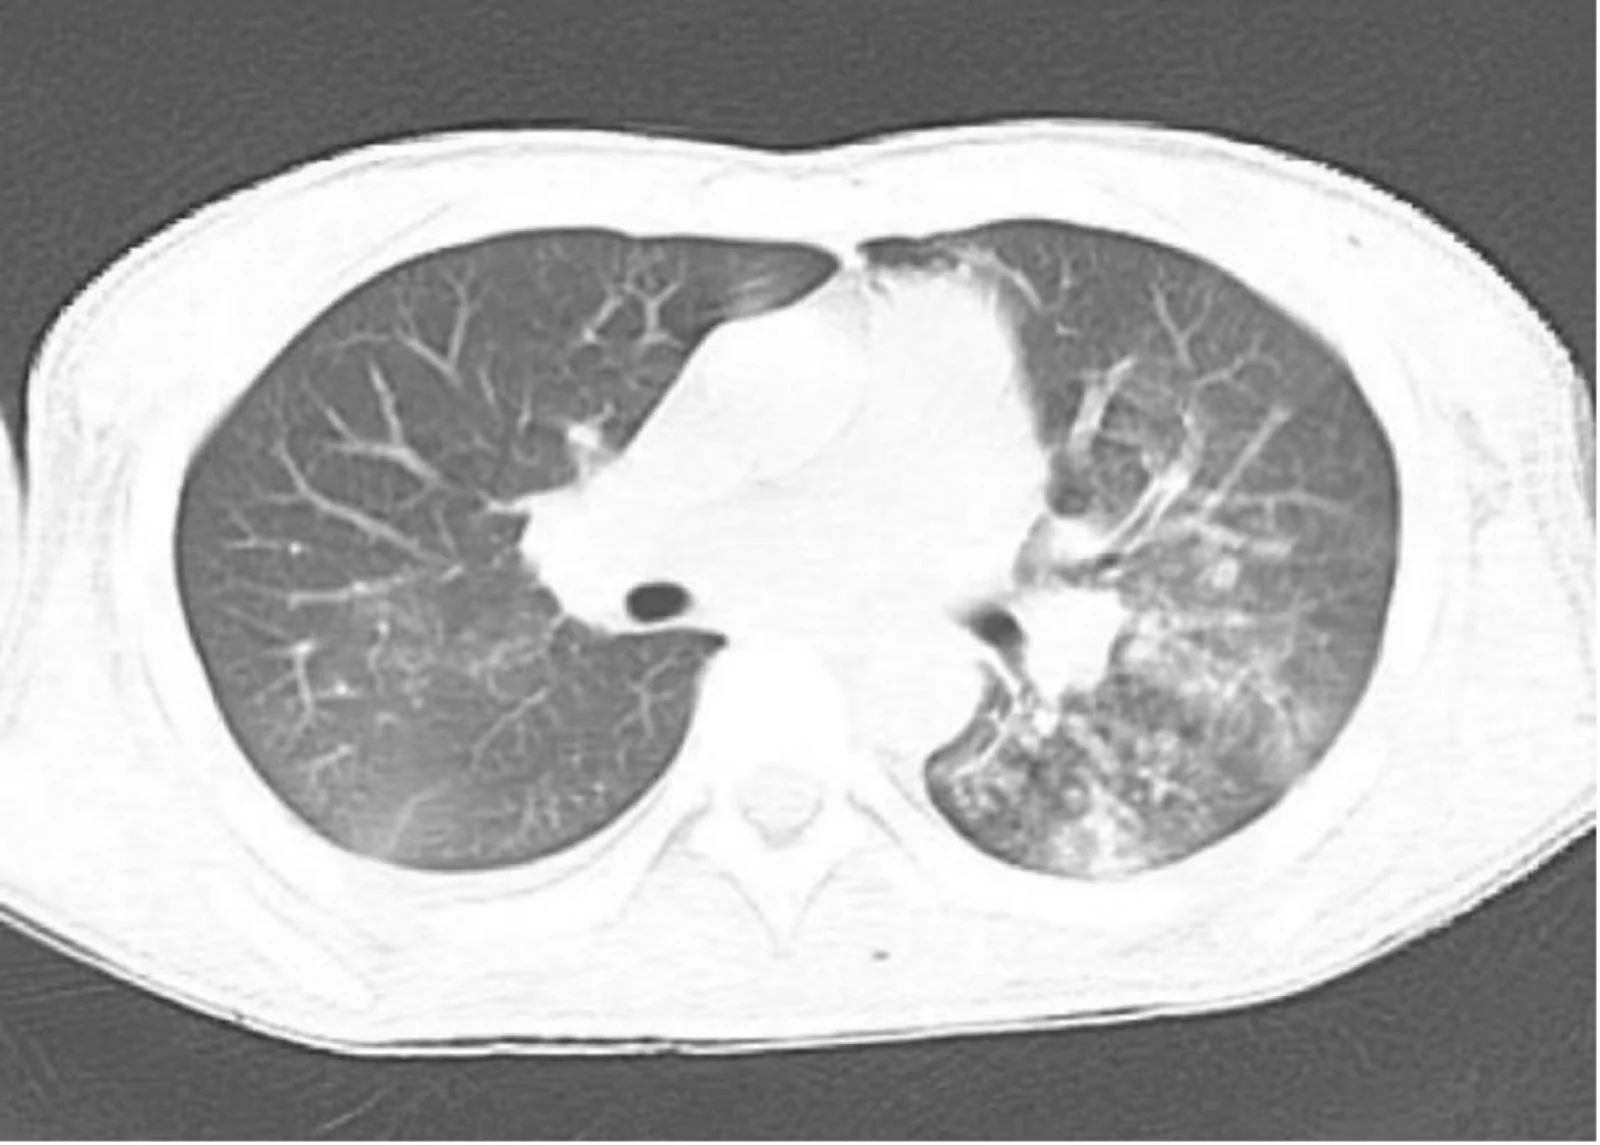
A repeat CT performed 5 days after treatment demonstrated significant improvement in pulmonary edema with complete resolution of the subcutaneous, mediastinal, and subpleural gases. CT = computed tomography.
